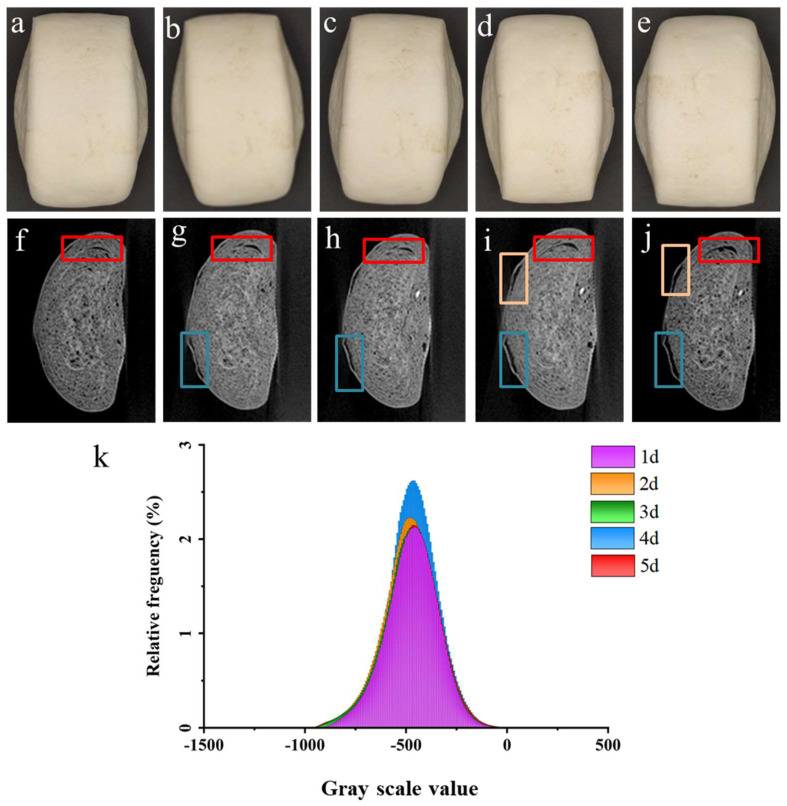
(a–e) Visual images, (f–j) X-ray CT images and (k) GSV histograms of CSBs during storage (1d, 2d, 3d, 4d and 5d).
